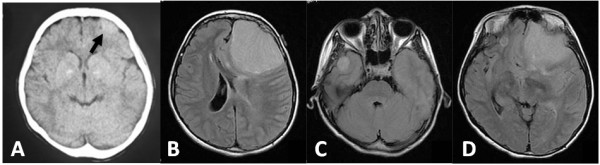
Preoperative axial computed tomography (CT) and fluid-attenuated inversion recovery (FLAIR) magnetic resonance imaging (MRI). (A) CT image showing a tumor approximately 1.5 cm in diameter in the left frontal convexity (arrow). (B) Tumor in the left frontal convexity. The tumor mass and perifocal edema caused a shift of the brain to the right side. (C) Tumor in the right middle cranial fossa. (D) Right frontal base tumor (cranial fossa) and the tumor in the left frontal convexity.
